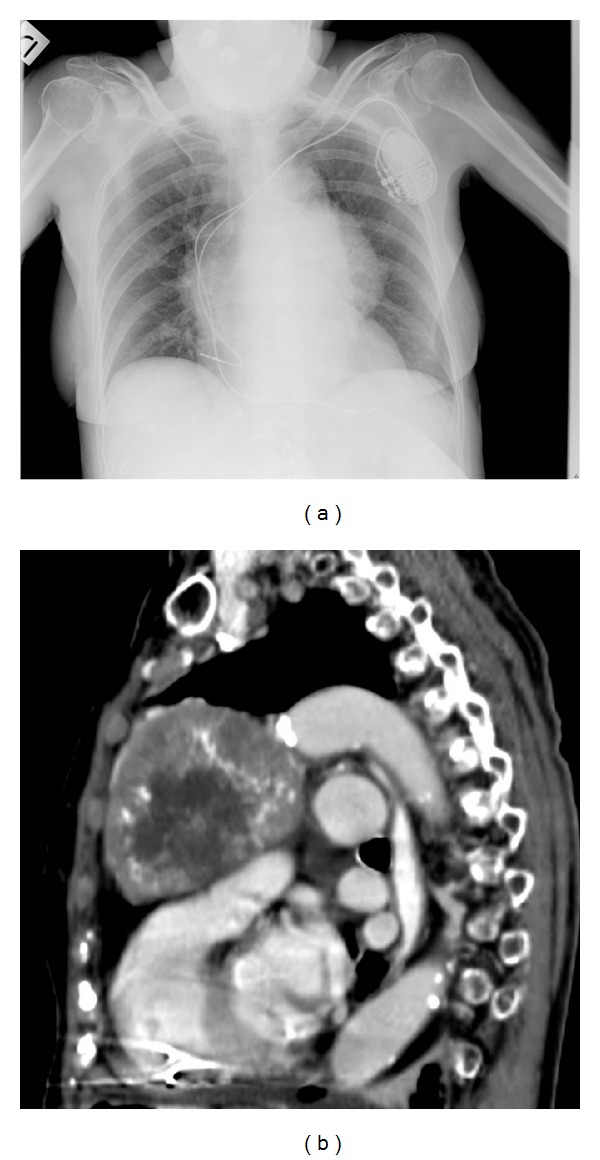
(a) Chest X-ray shows enlargement of the mediastinum. (b) Chest computed tomography image demonstrates a large inhomogeneous mass with calcifications in the anterior mediastinal compartment. The tumor is in close relationship with the ascending aorta and the common trunk of the pulmonary artery, with apparent preservation of a cleavage plane.
